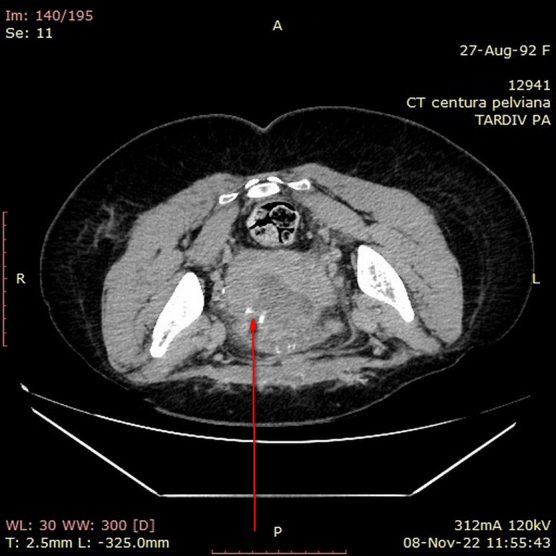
Contrast passing through the two cavities (CT, axial plane). CT, computed tomography.
